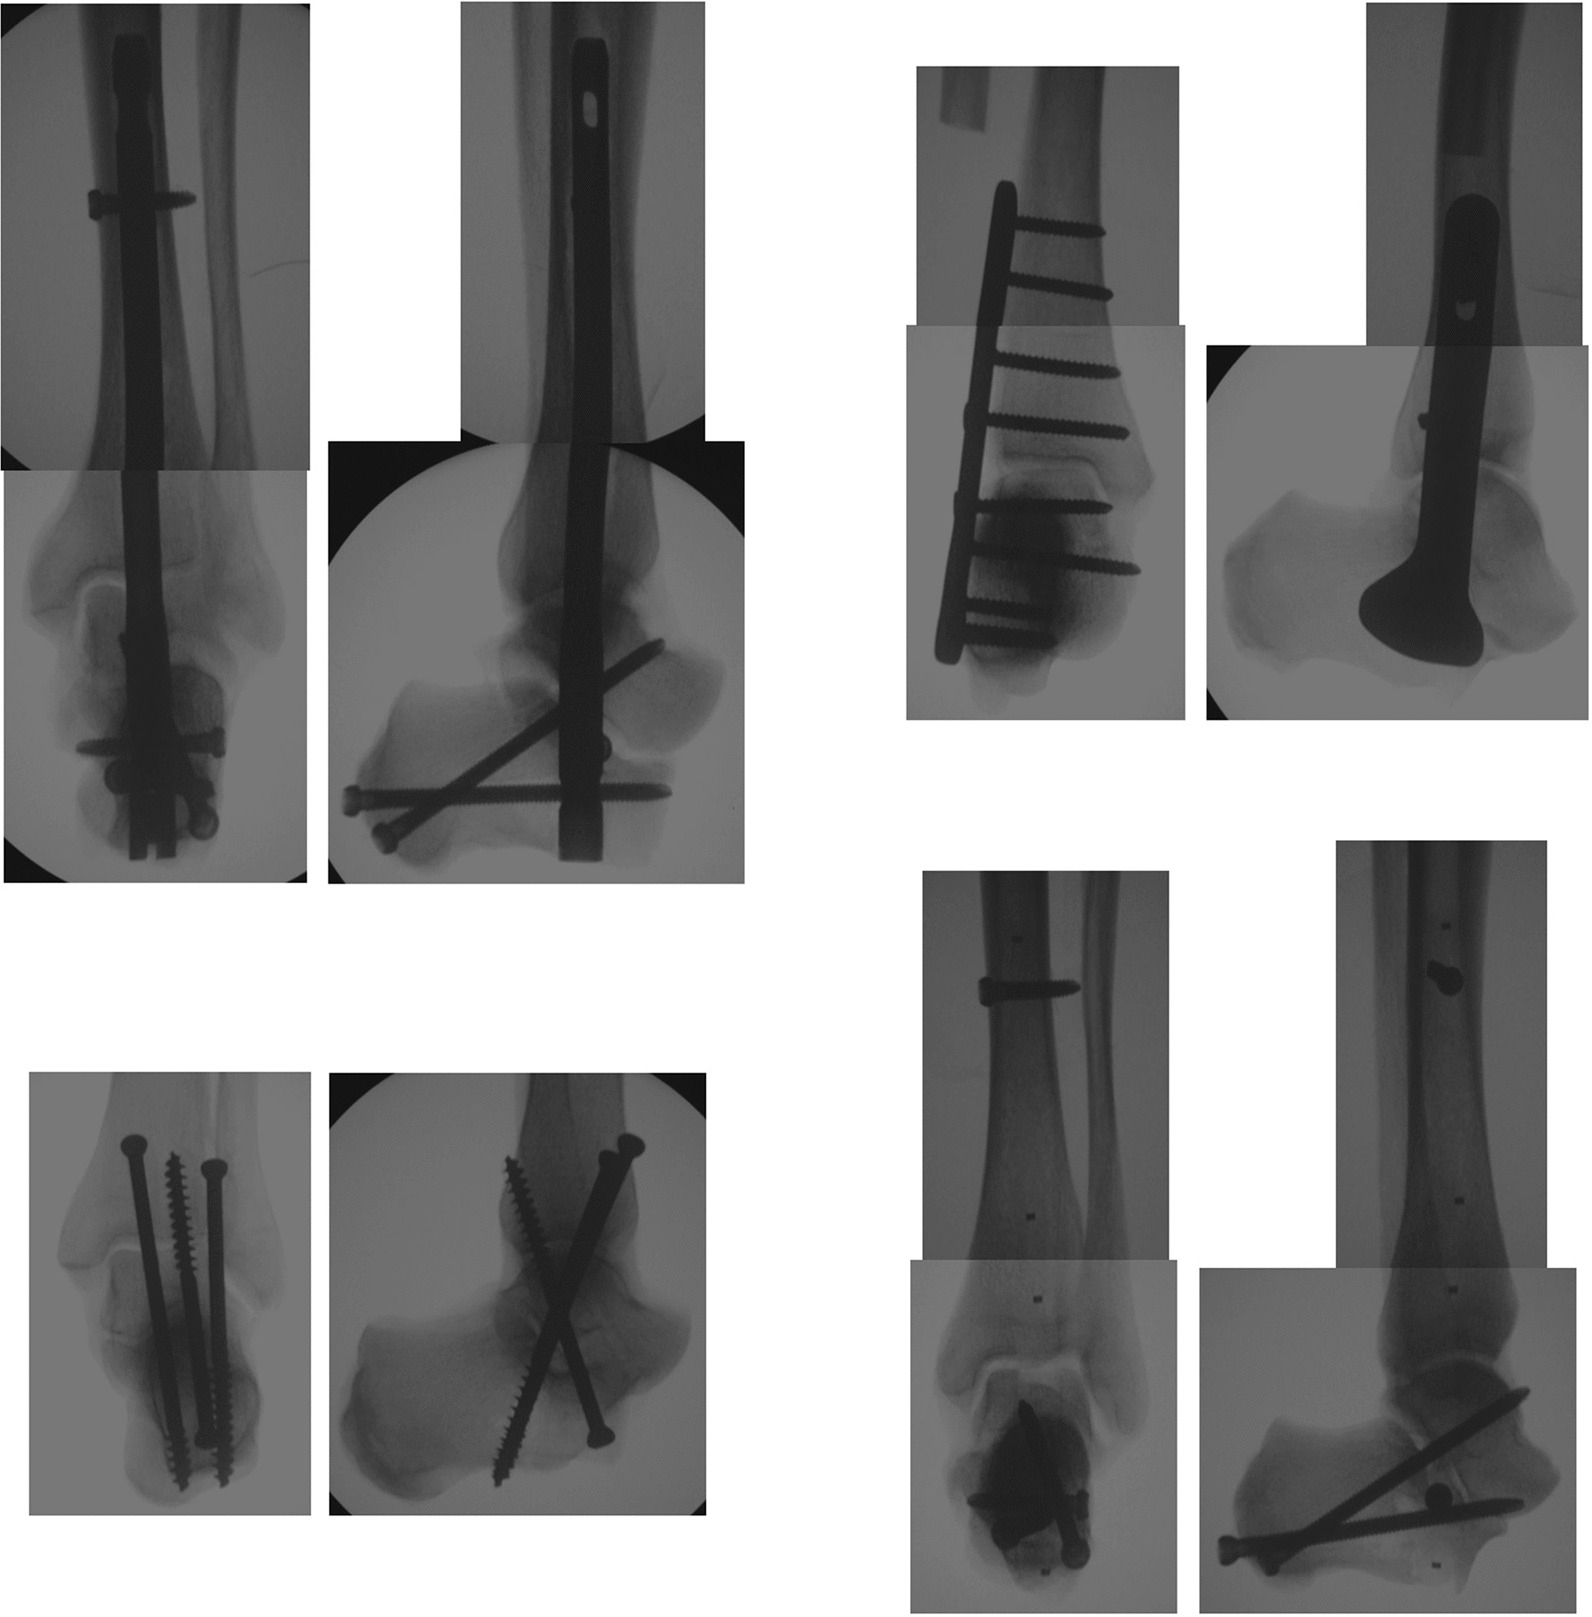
Radiographs in anteroposterior and lateral projections after tibiotalocalcaneal arthrodesis with nail, plate, screws, or the bone stabilization system
